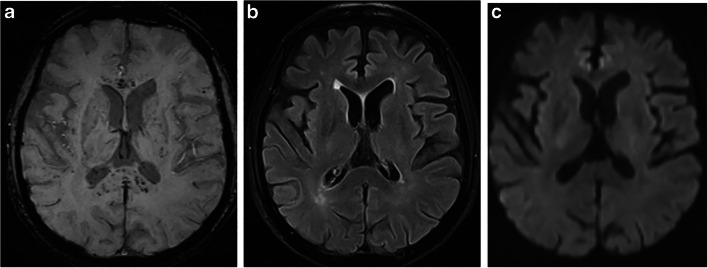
In a 54-year-old male liver transplant patient with respiratory failure and mechanical ventilation, SWI (a) shows numerous microsusceptibilities in the corpus callosum (type III). Axial fluid-attenuated inversion-recovery (FLAIR) and diffusion-weighted (DWI) images (c) do not show any abnormality
